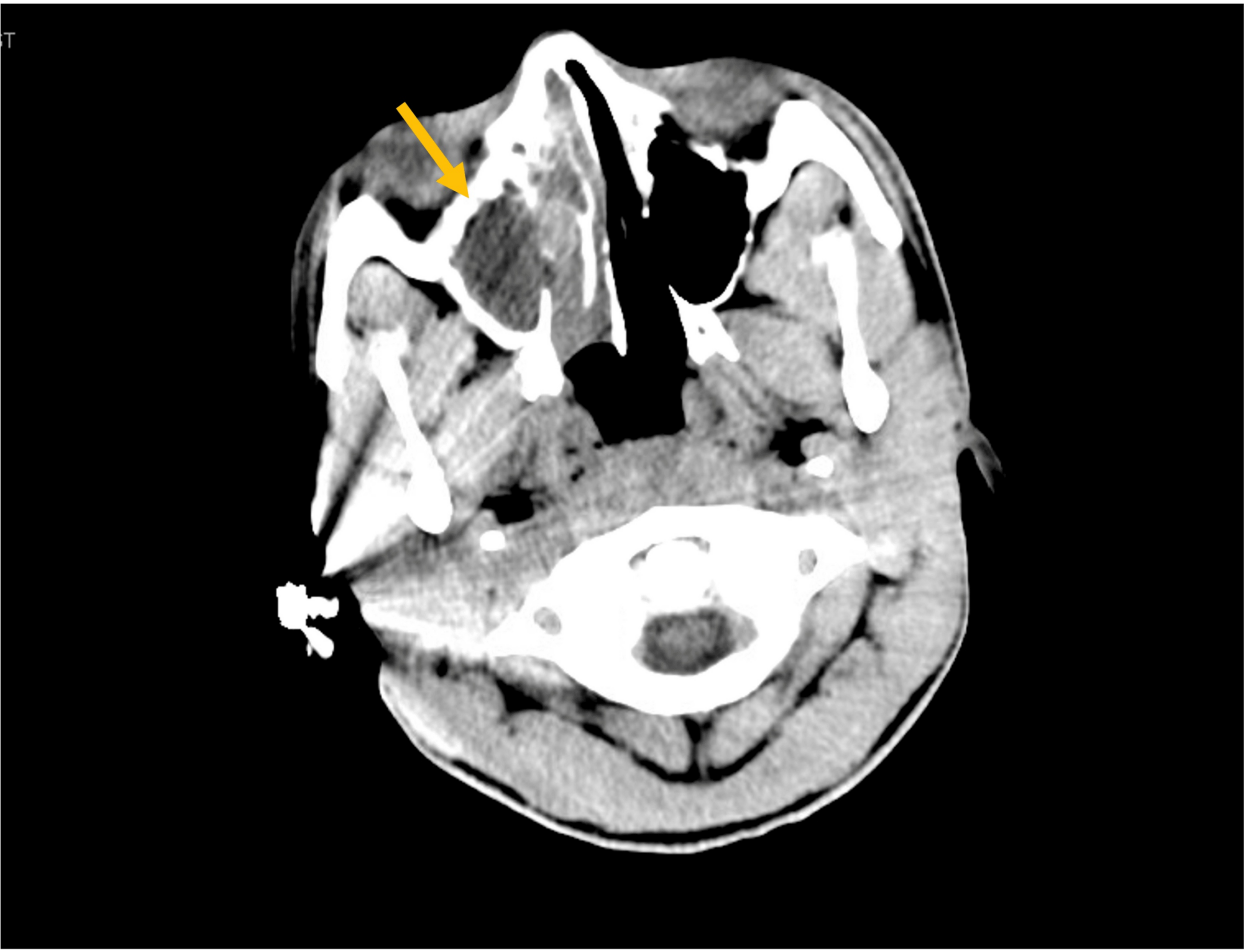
Non-contrast CT scan showed a large degree of opacification with what appears to be expansion suggestive of a mucocele involving the right frontal, right ethmoid and right maxillary sinuses.
